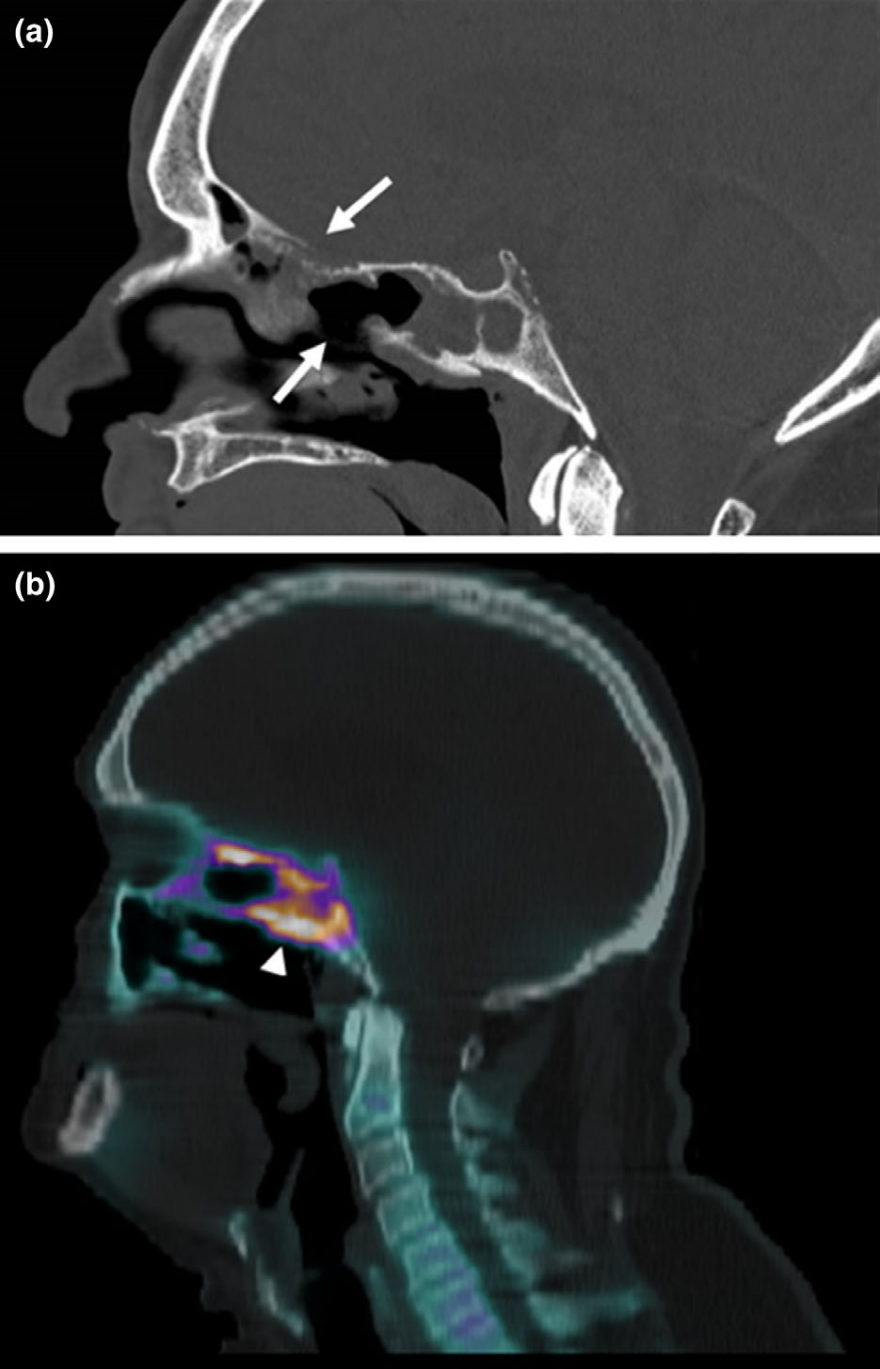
Non‐contrast sagittal reformat of CT paranasal sinuses (a) of a 72‐year‐old male patient with GPA showing bony destruction and osteomyelitis of the ethmoid and sphenoid bones (arrows). Focal hyperaemia and increased osteoblastic activity centred in the sphenoid bone extending to the sella turcica and clivus were present on the nuclear medicine bone scan (arrowhead) (b).
